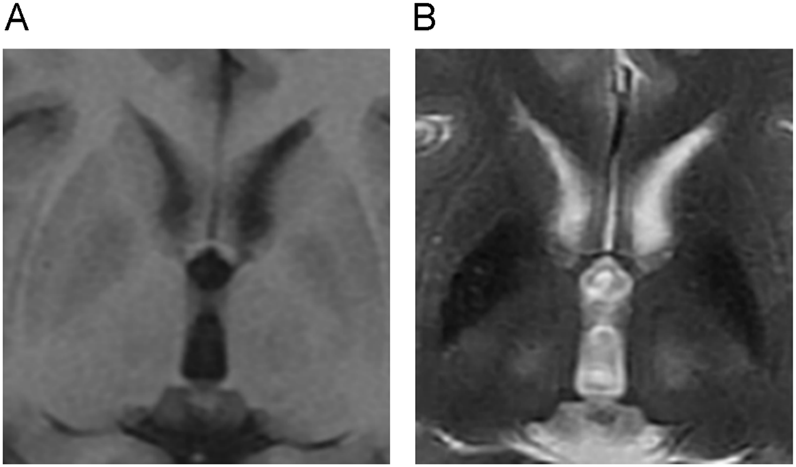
Conventional MRI phase images of WD patients. Many patients showed hypointense areas on T1-weighted images(A) and hyperintense areas on T2-weighted images(B).
